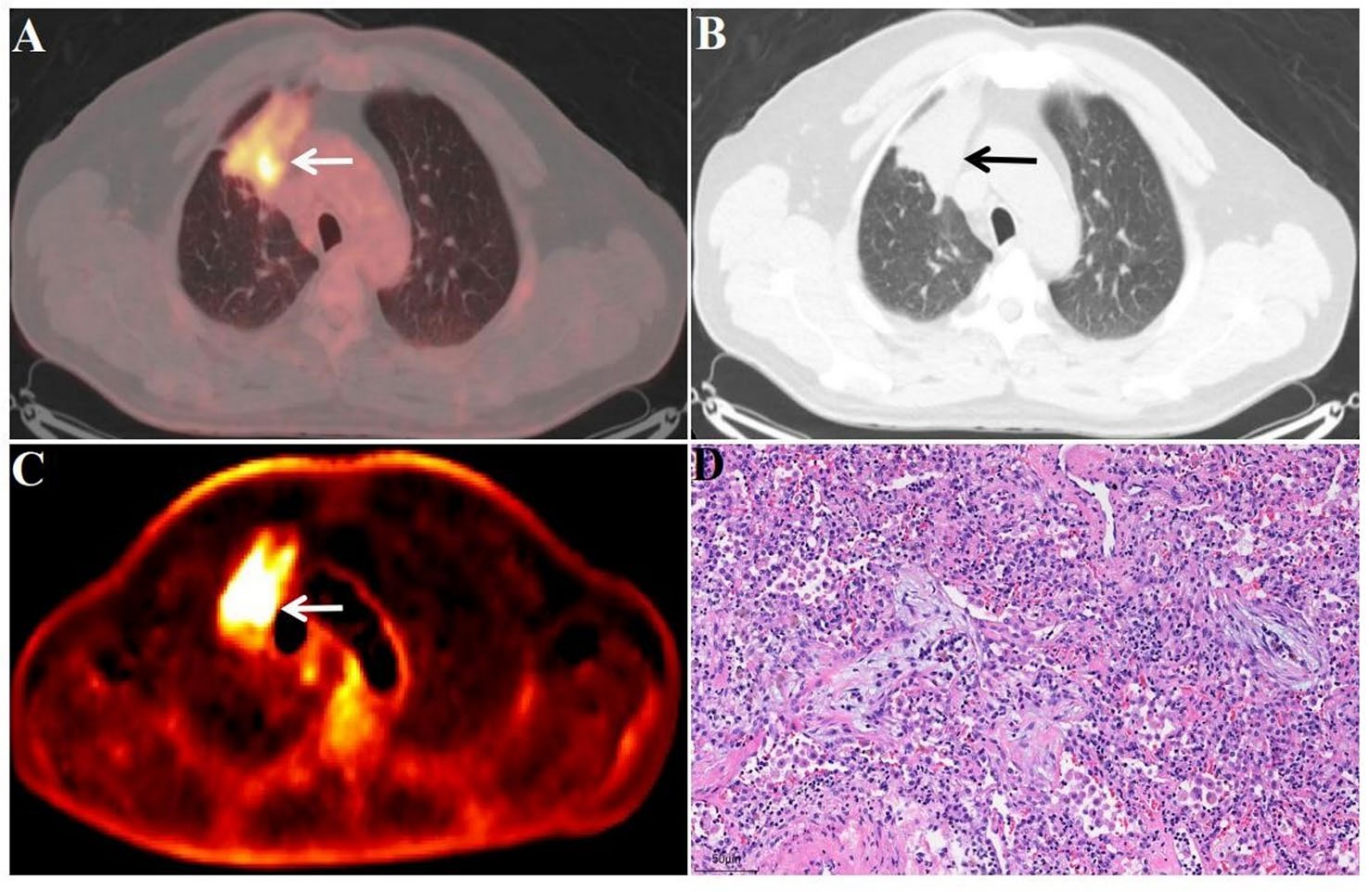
FDG-PET/CT images of a benign lesion A 59-year-old male patient. Surgical pathology confirmed an inflammatory lesion (D, 20x field of view) in the upper lobe of the right lung (white/black arrow), with a size of 5.3 × 4.5 cm (A and B, white/black arrow), SUV_max_ of 7.4 (A, white arrow), and K_i_ of 0.0120 ml/g/min (C, white arrow)
